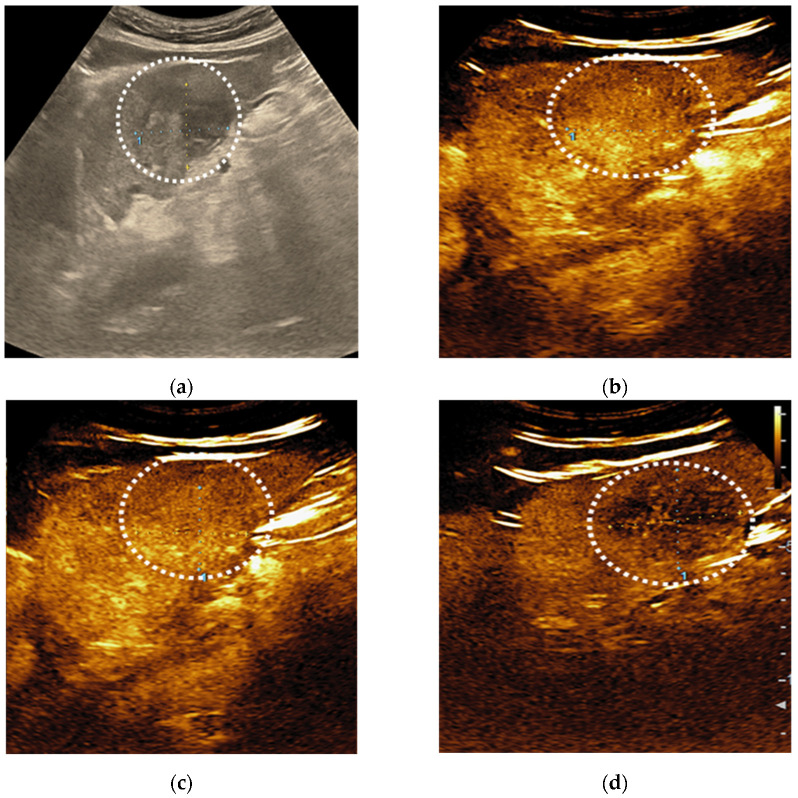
Hepatocellular carcinoma detected in a 54-year-old man with alcoholic cirrhosis, characterised as LI-RADS-5 on CEUS. On B mode ultrasound is observed an inhomogeneous lesion in the right liver lobe (a). On CEUS, the liver lesion shows an arterial phase enhancement (b) followed by washout appearance that was mild in degree and late in onset (c,d).
